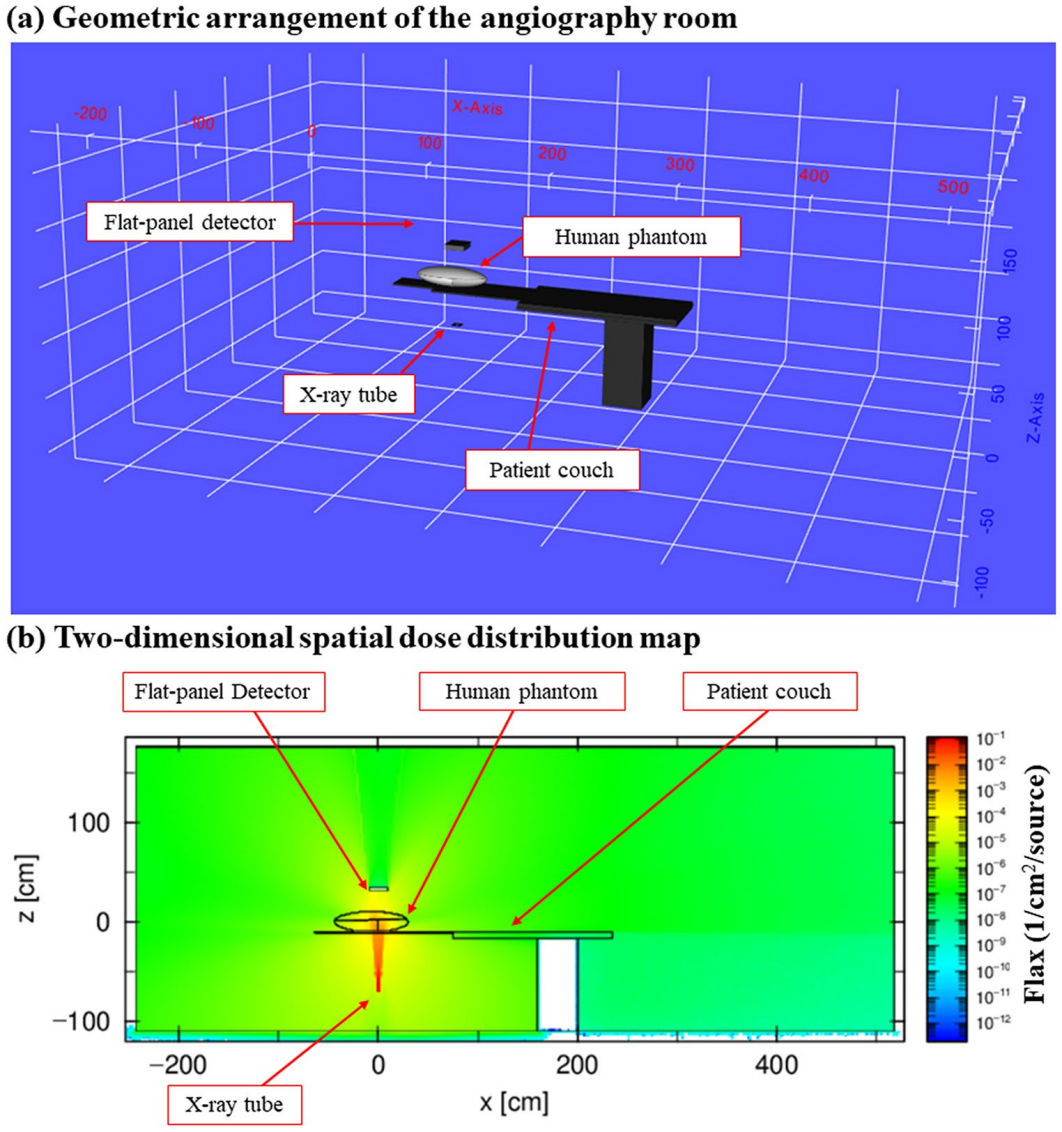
Geometry of the angiography room reproduced by (a) PHITS and (b) the two-dimensional spatial dose distribution. The reference (0) of the coordinate system is the long-axis direction of the patient couch, the X-ray tube source position is the short-axis direction, and the height direction is the isocenter
